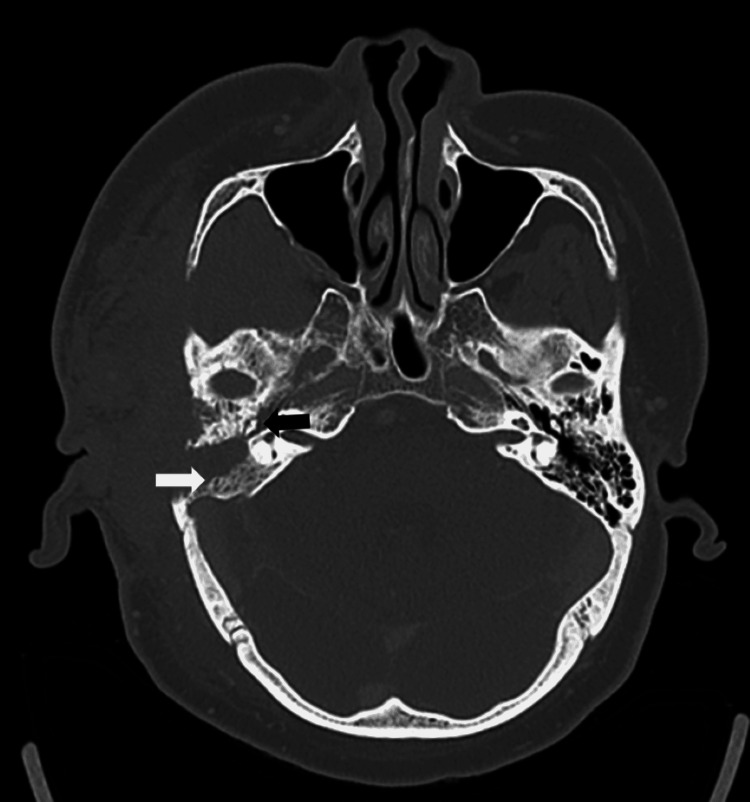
Axial HRCT of the temporal bone showing soft tissue density in right middle ear occupying epitympanum (black arrow). Bony erosion at anterior the wall of right mastoid air cells, fluid-filled right mastoid air cells (white arrow). HRCT: High resolution computed tomography
